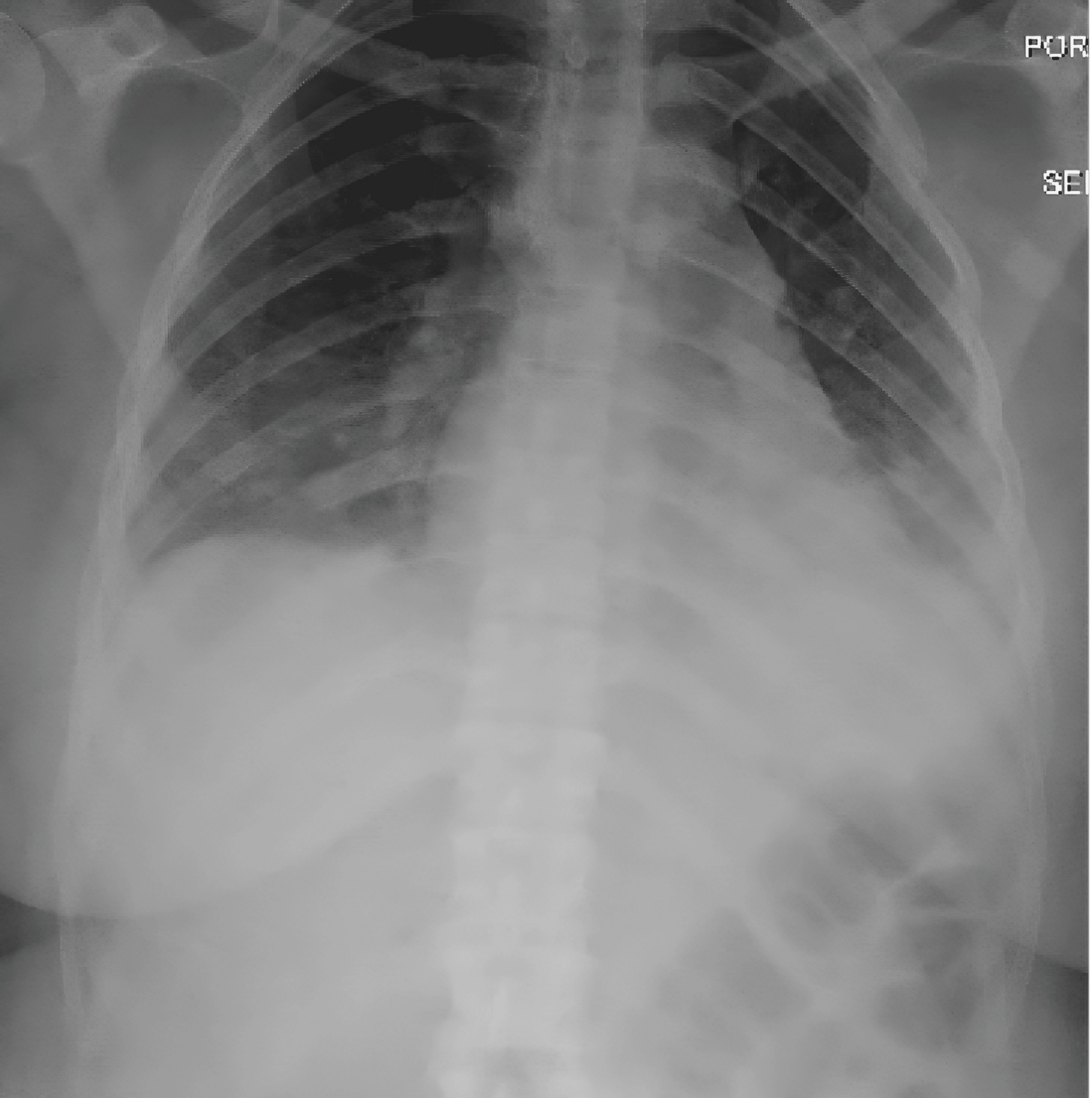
Portable chest X-ray: AP view. Persistent basilar opacities may be related to atelectasis or pneumonia. There is pulmonary vascular congestion, and no pleural effusion or cardiomegaly.
